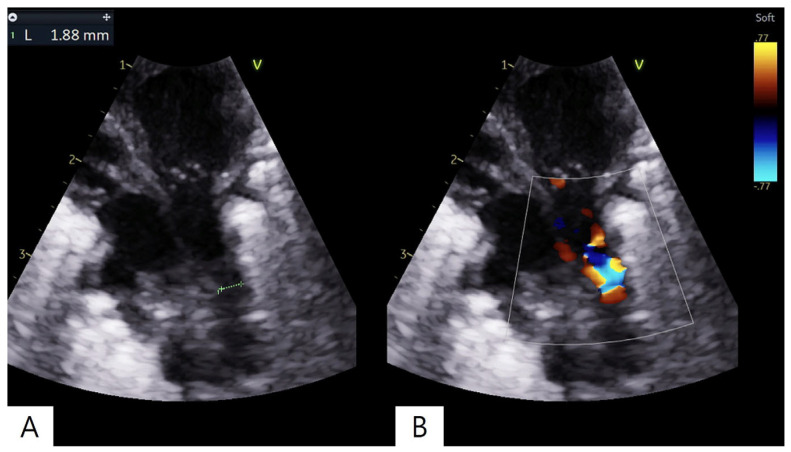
Transthoracic echocardiographic image obtained on postnatal day 8 demonstrating a hemodynamically significant patent ductus arteriosus. (A) Two-dimensional echocardiography shows a ductal diameter of approximately 1.8 mm. (B) Color Doppler imaging demonstrates bidirectional shunt flow across the ductus arteriosus. Numbers 1–3 on the left margin indicate depth markers of the ultrasound image, and the dotted green line represents the measured ductal diameter.
